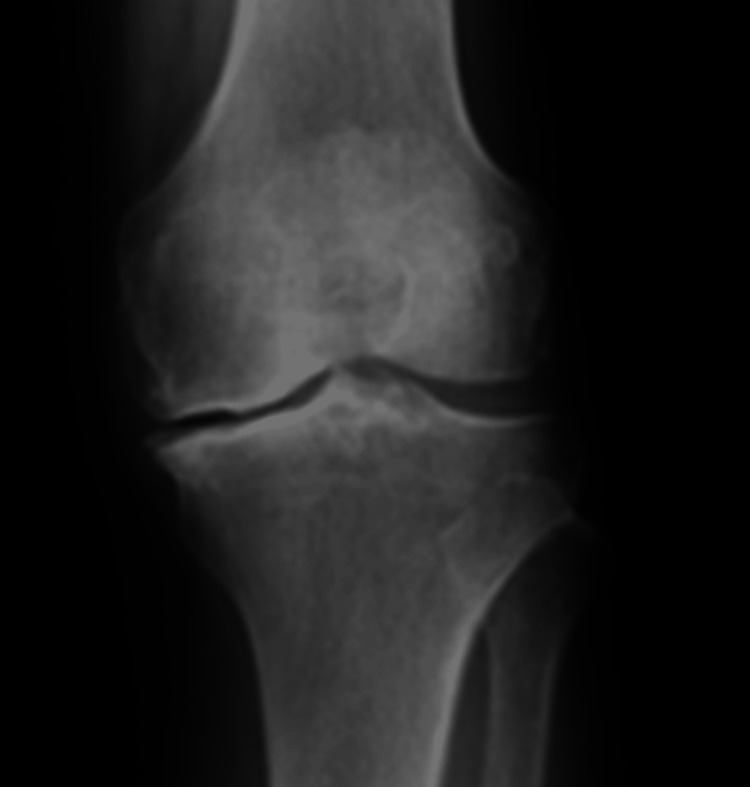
Preoperative radiography
